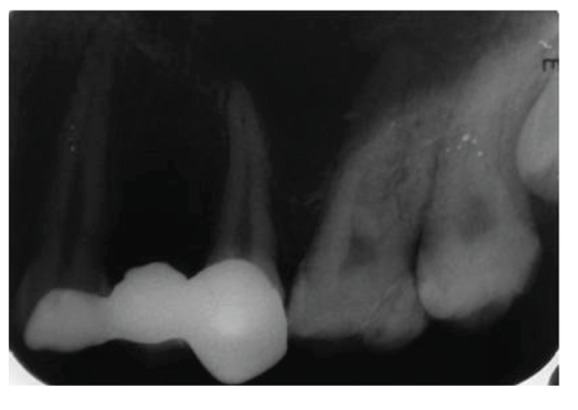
Intraoral periapical radiograph with upper left posterior region at a 2-year followup.
